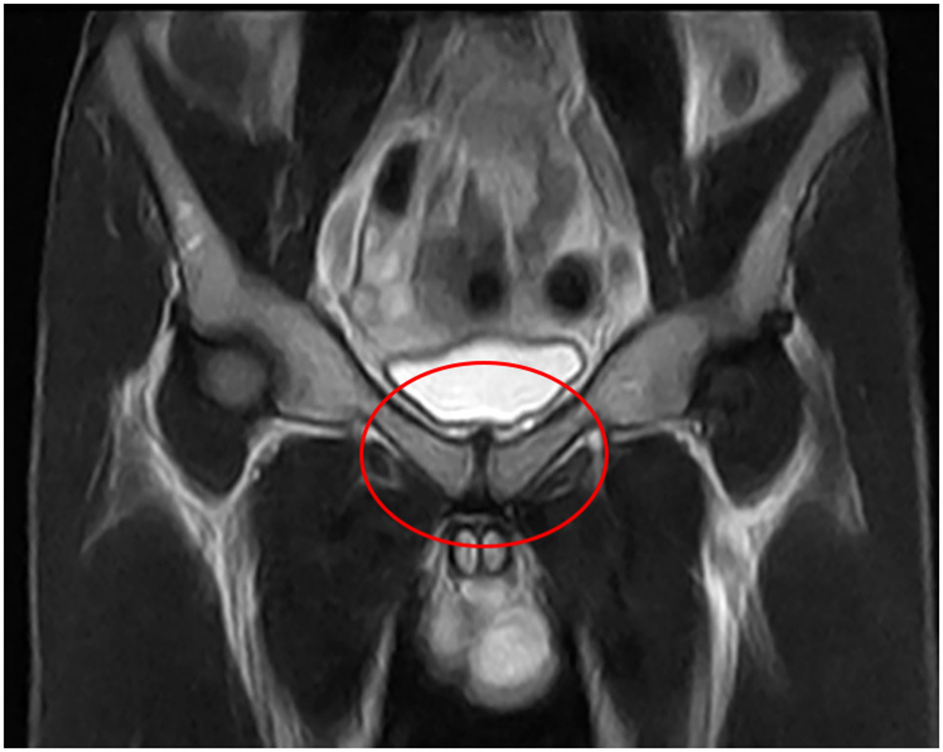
MRI investigation of the frontal plane in T2W, in which no functional overload of the pubic area is present.
